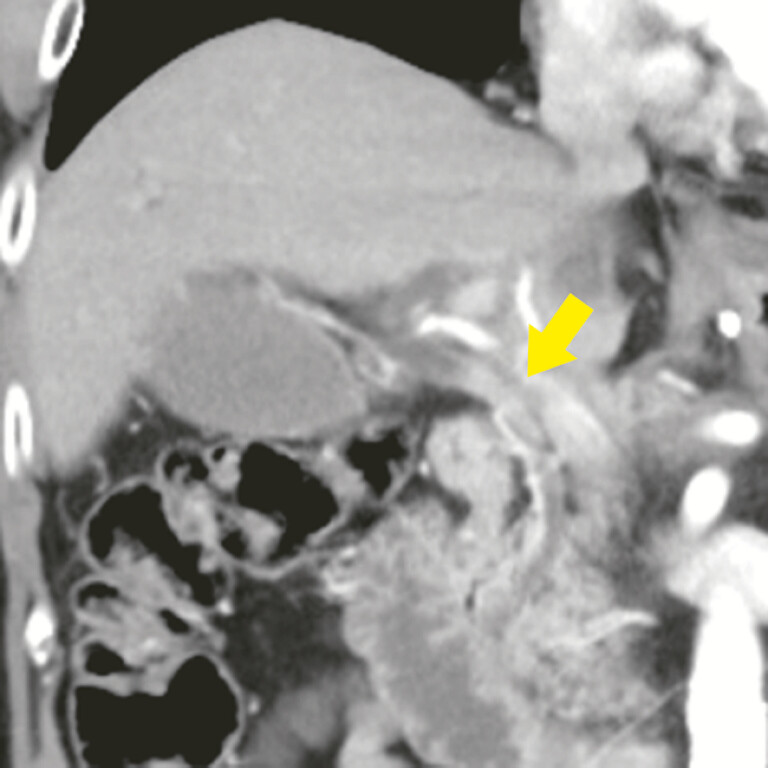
Contrast-enhanced computed tomography in a patient with a history of gastric cancer treated with distal gastrectomy and Roux-en-Y reconstruction revealed mild thickening and stricture (arrow) of the common bile duct wall and upstream biliary dilation.
